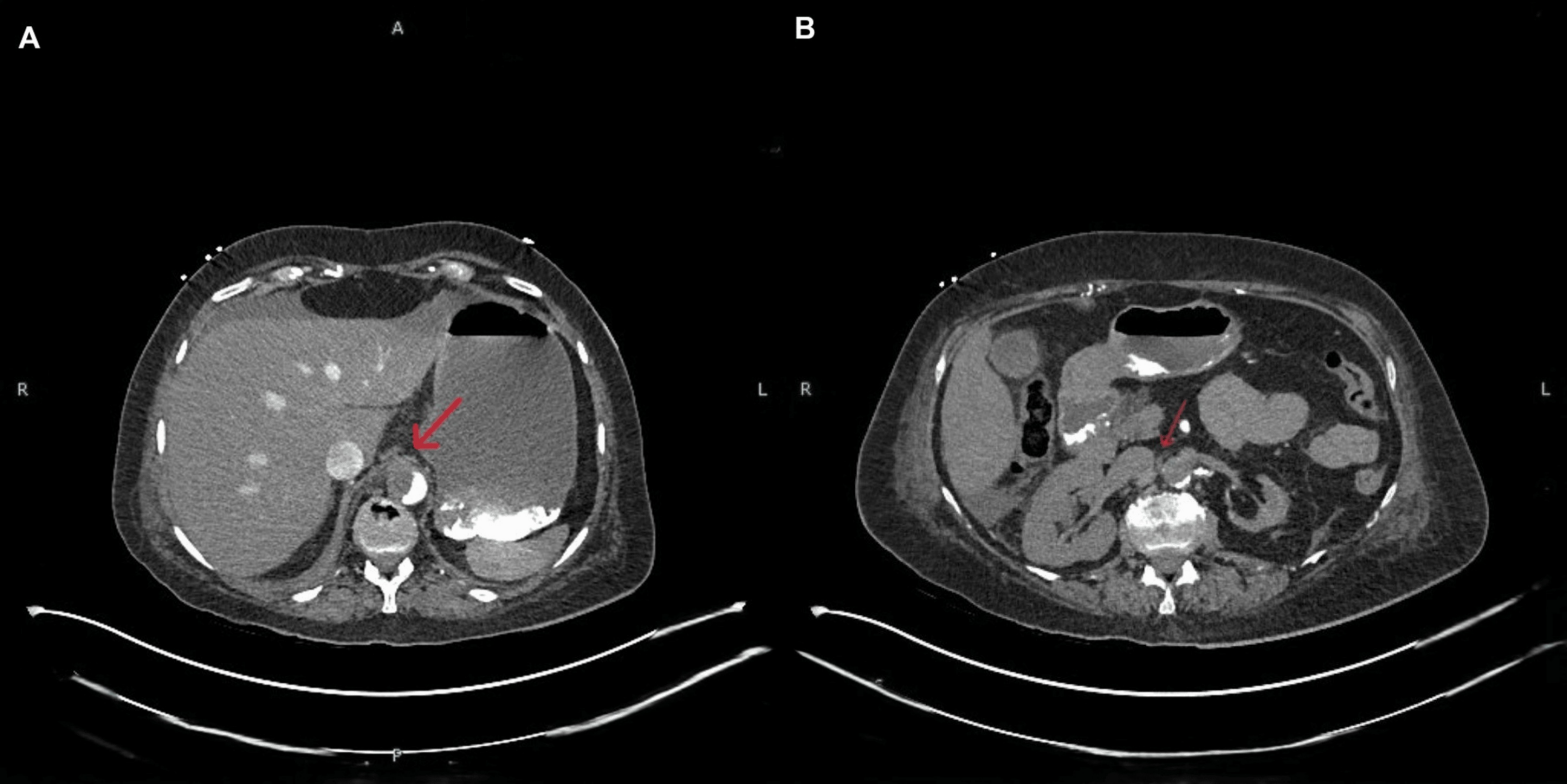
Computed tomography (CT) of the abdomen showing an atheromatous dissection and occlusion of the abdominal aorta below the level of the superior mesenteric artery (SMA), with the complete occlusion of the right renal artery due to the acute thrombosis and near-total infarction of the right renal parenchyma. The left kidney appears markedly atrophic with chronic ischemic changes, attributable to long-standing proximal left renal artery occlusion.
